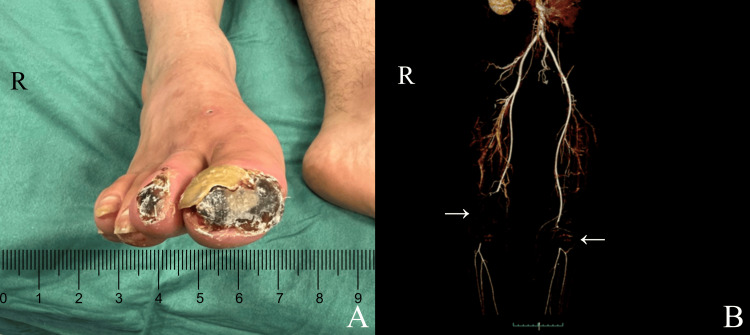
Ischemic ulcers in distal portion of right I-II toe (A). Posterior view of a three-dimensional reconstruction of a lower extremity contrast-enhanced CT arteriogram revealing obstruction of the right superficial femoral artery up to the level of the popliteal artery (A) as well as occlusion of the left popliteal artery (B).
